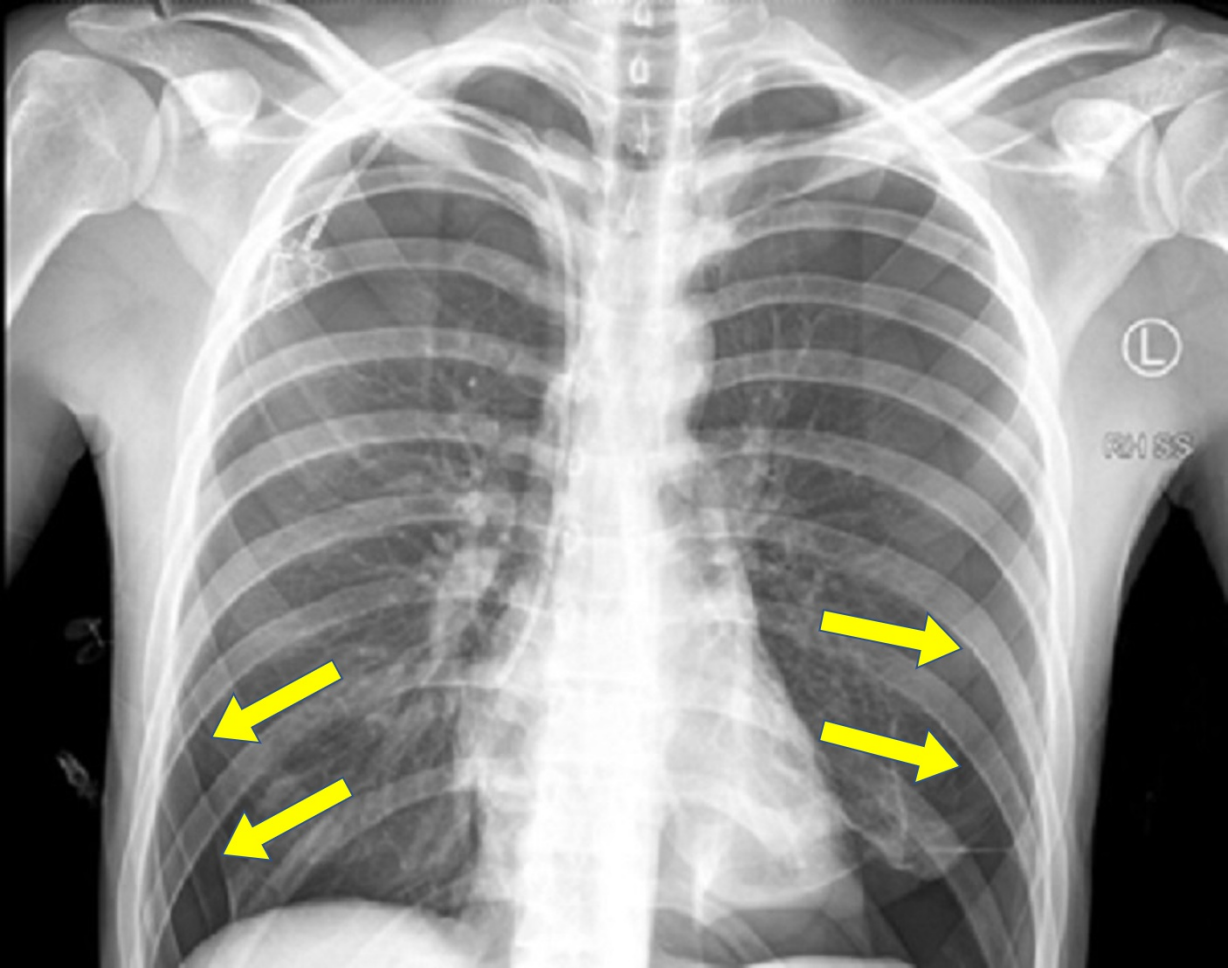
The chest x-ray showing large bilateral pneumothoraces.
